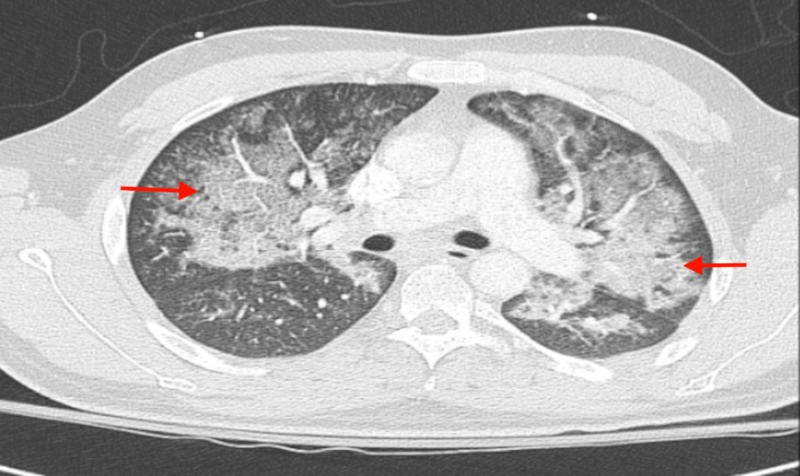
CT angiogram of the chest showing extensive ground glass opacity in both lungs mostly sparing the periphery.
